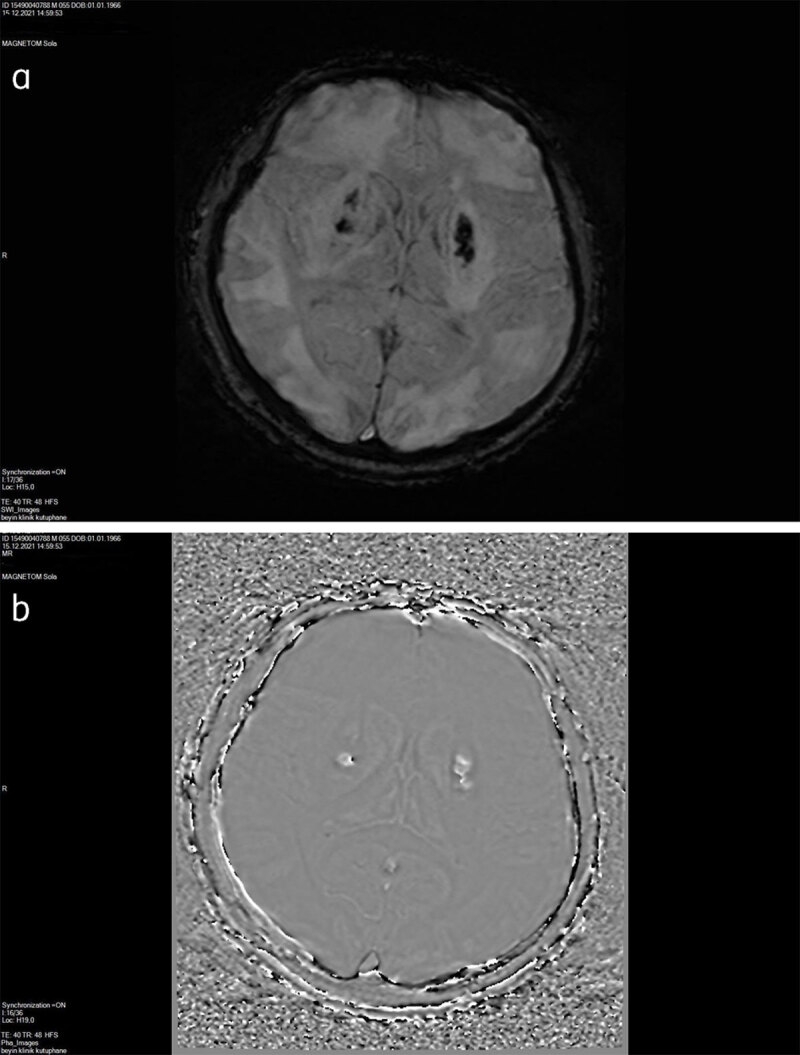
a Magnitude SWI demonstrated hypointense hemorrhagic foci on both putaminal regions. b Phase-contrast SWI reveals opposite signal intensity due to positive shift effect of deoxyhemoglobin on left-handed MRI system.
